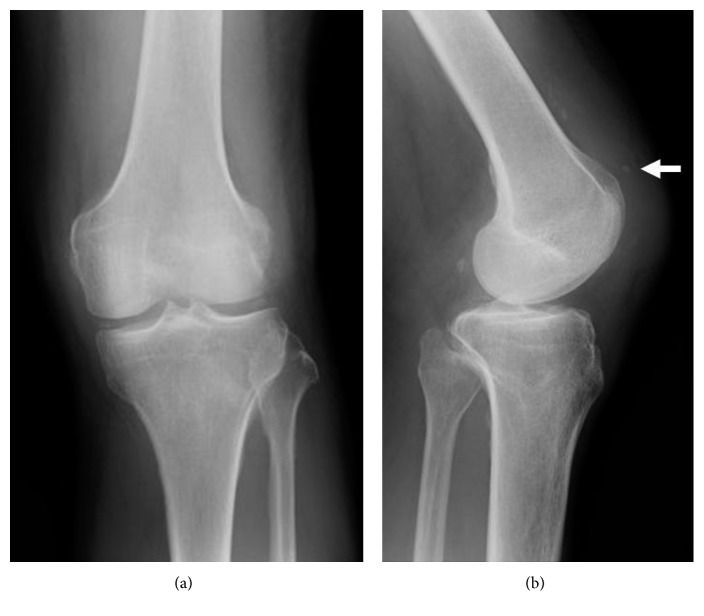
Plain radiographic anteroposterior view (a) and lateral view (b) of the left knee showing the absence of a patella and the presence of a small heterotopic calcification (white arrow) at the distal side of the quadriceps tendon.
